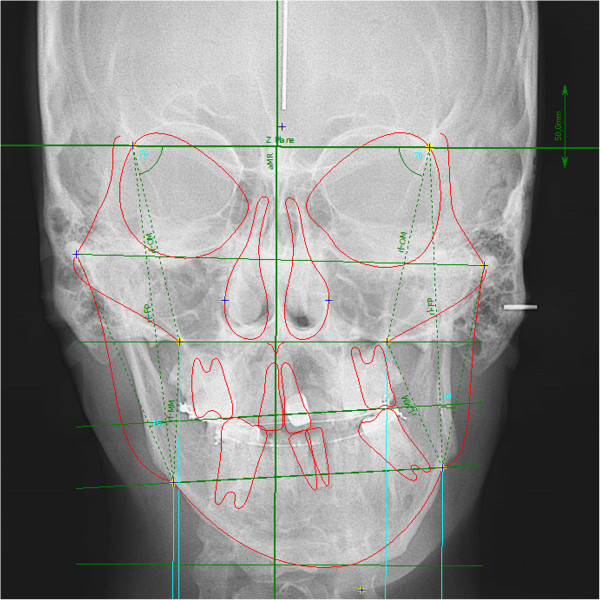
Presurgery frontal cephalometric x-ray shows the compensatory transverse canting of the maxillary occlusal plane.
